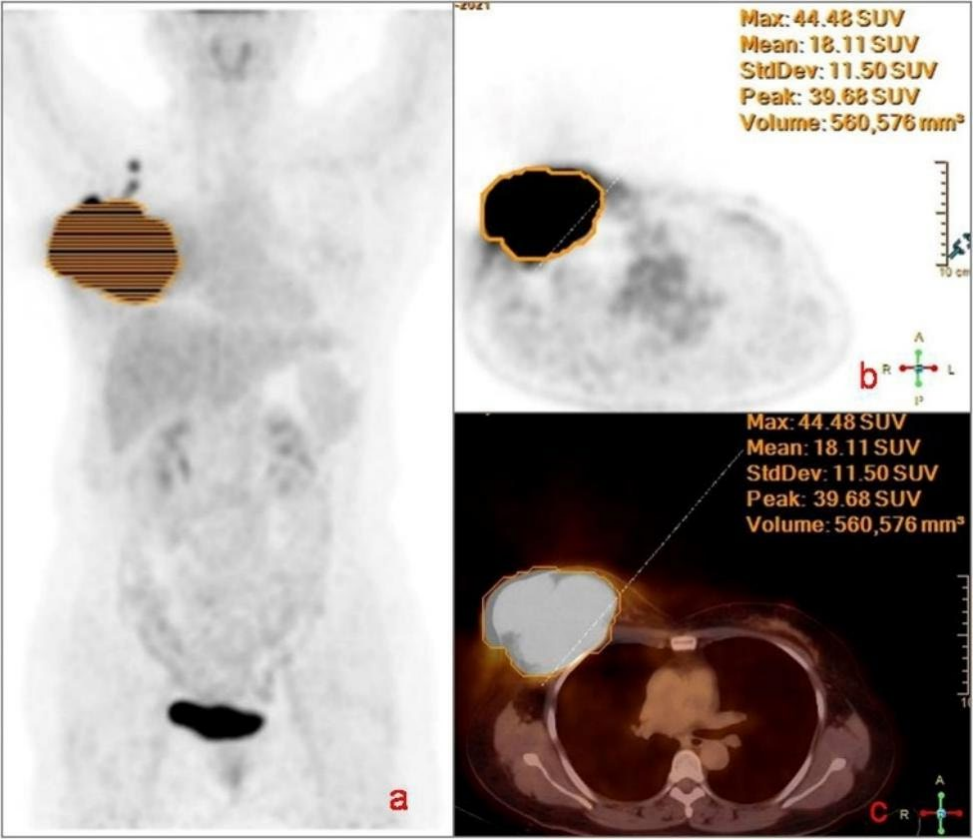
Metabolic parameters measurements of PET/CT scan. Female, 56 years-old, with BC. MIP image (a) showed the intense uptake of the BC lesion at the right side. The 3D VOI was delineated by physician with the rule of 40% of the maximum standardized uptake value (SUVmax) on the PET image (b) or PET/CT fusion images (c). SUVmax, SUVmean, SD, MTV were measured respectively
